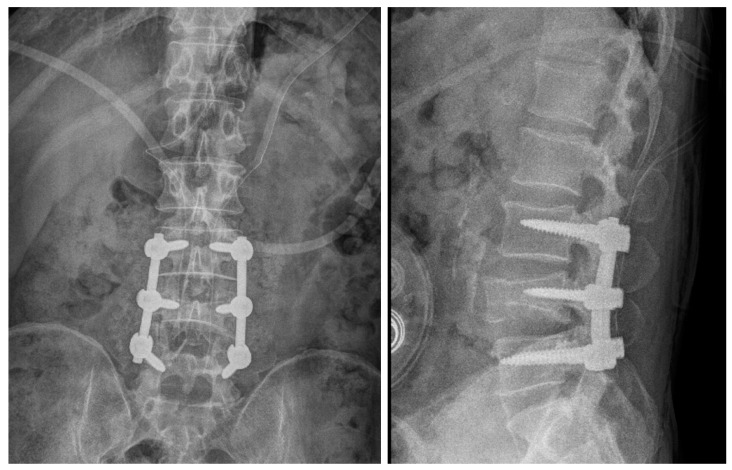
Radiographic image after the first surgery showing L3/L4/L5 fusion state.
